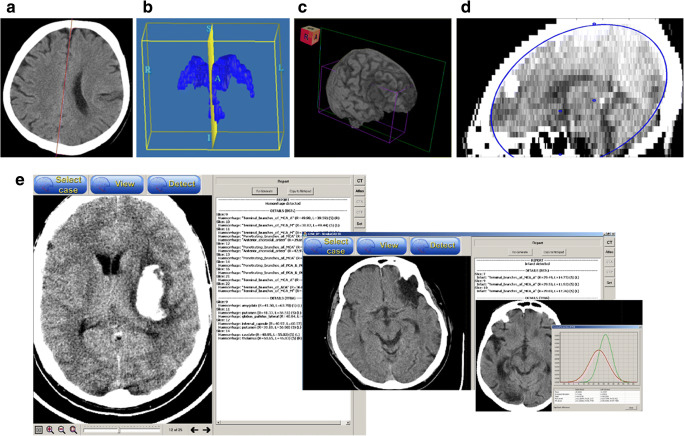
Atlas-assisted stroke diagnosis in the emergency room: a) midsagittal line extracted on a sample axial unenhanced CT image; b) ventricular system segmented in 3D along with the midsagittal plane; c) extracted brain in 3D that is volume rendered with a clearly discernible ischemic infarct; d) atlas to CT scan mapping by means of the ellipse-fitting method (the positions of the anterior and posterior commissures are also marked on this scan having a high slice thickness); and e) results of atlas-assisted analysis: left) hemorrhage detection (an axial image with a hemorrhage is shown on the left with the report for the whole brain on the right containing the details regarding the numbers of the hemorrhagic slices, affected blood supply territories (top) and anatomic structures (bottom) along with the corresponding intensity values (scaled to 8 bits) for the right and left hemispheres), center) infarct detection (an image with a part of the report), and right) left-right ROI intensity distribution curves with an axial image showing the infarcted areas (note a substantial mismatch in the corresponding curves in terms of their height, mean, and standard deviation)
